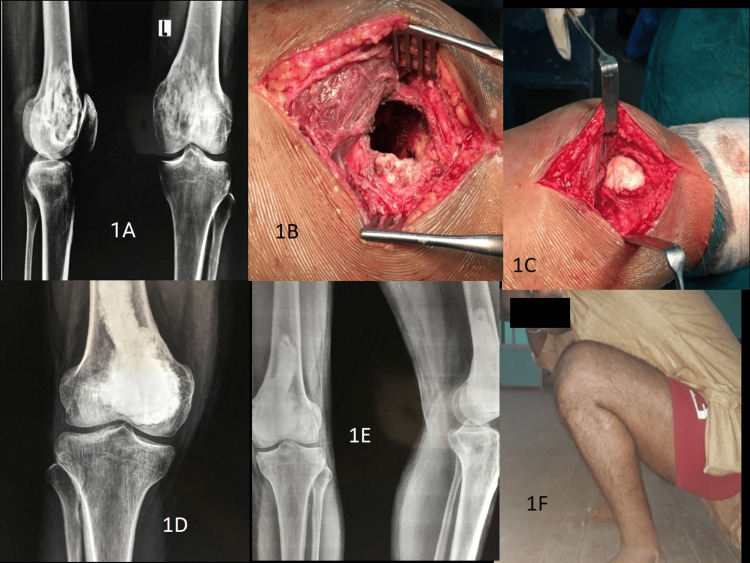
Recurrent GCT of distal femur in a 31-year-old male treated with extended curettage and bone cementing: (A) Preoperative radiograph showing recurrent GCT in distal femur; (B) Cavity after extended curettage; (C) Cavity filled with bone cement; (D) Postoperative radiograph showing extended curettage with bone cementing; (E) Follow-up radiograph after six months; (F) Functional outcome GCT: giant cell tumor
